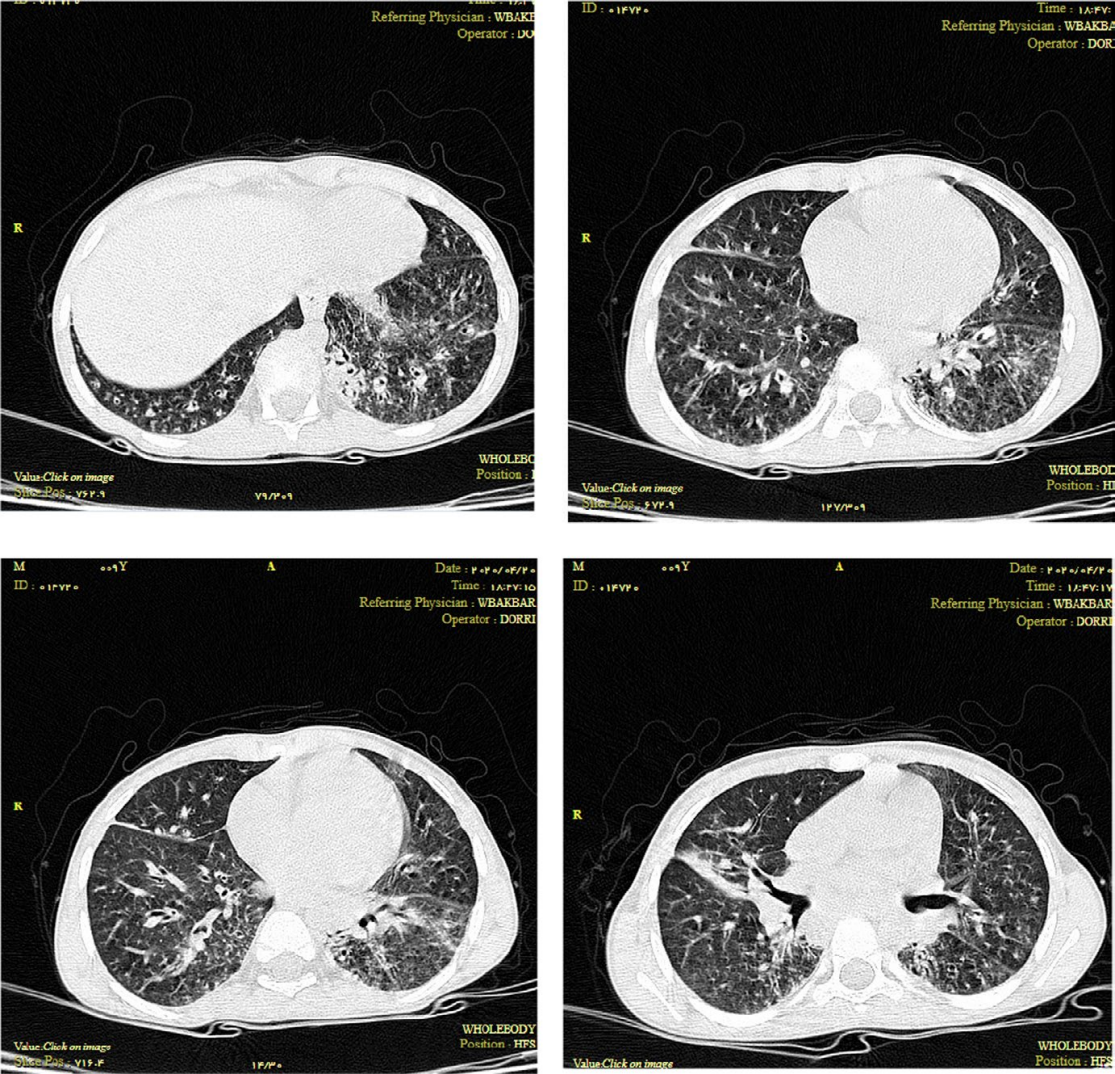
Computed tomography scan revealed evidence of bronchiectasis and collapse consolidations in an 8‐y‐old boy with primary antibody deficiency
